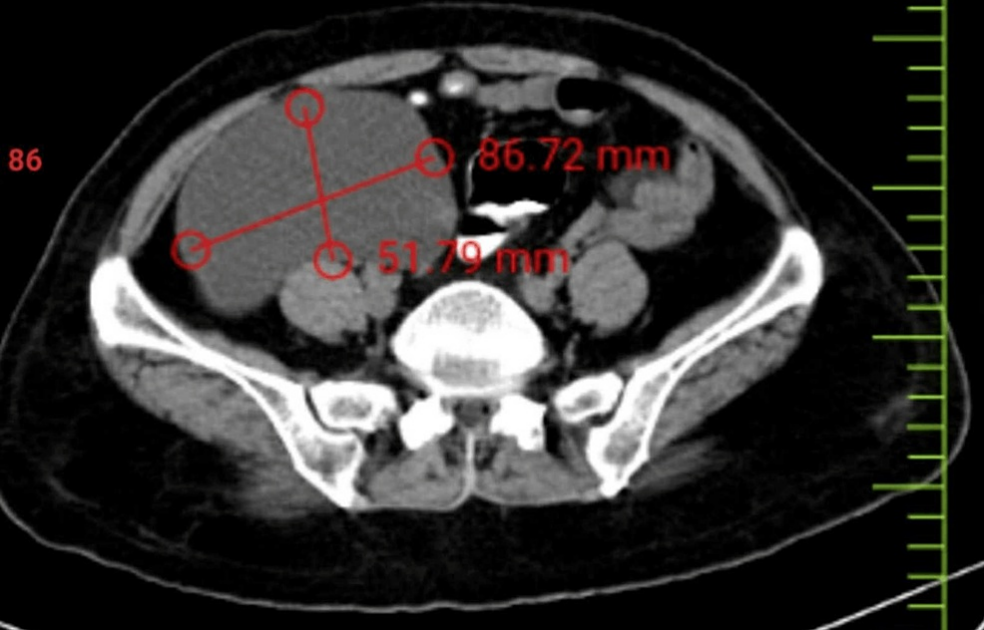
CT scan showing the mesenteric cyst
